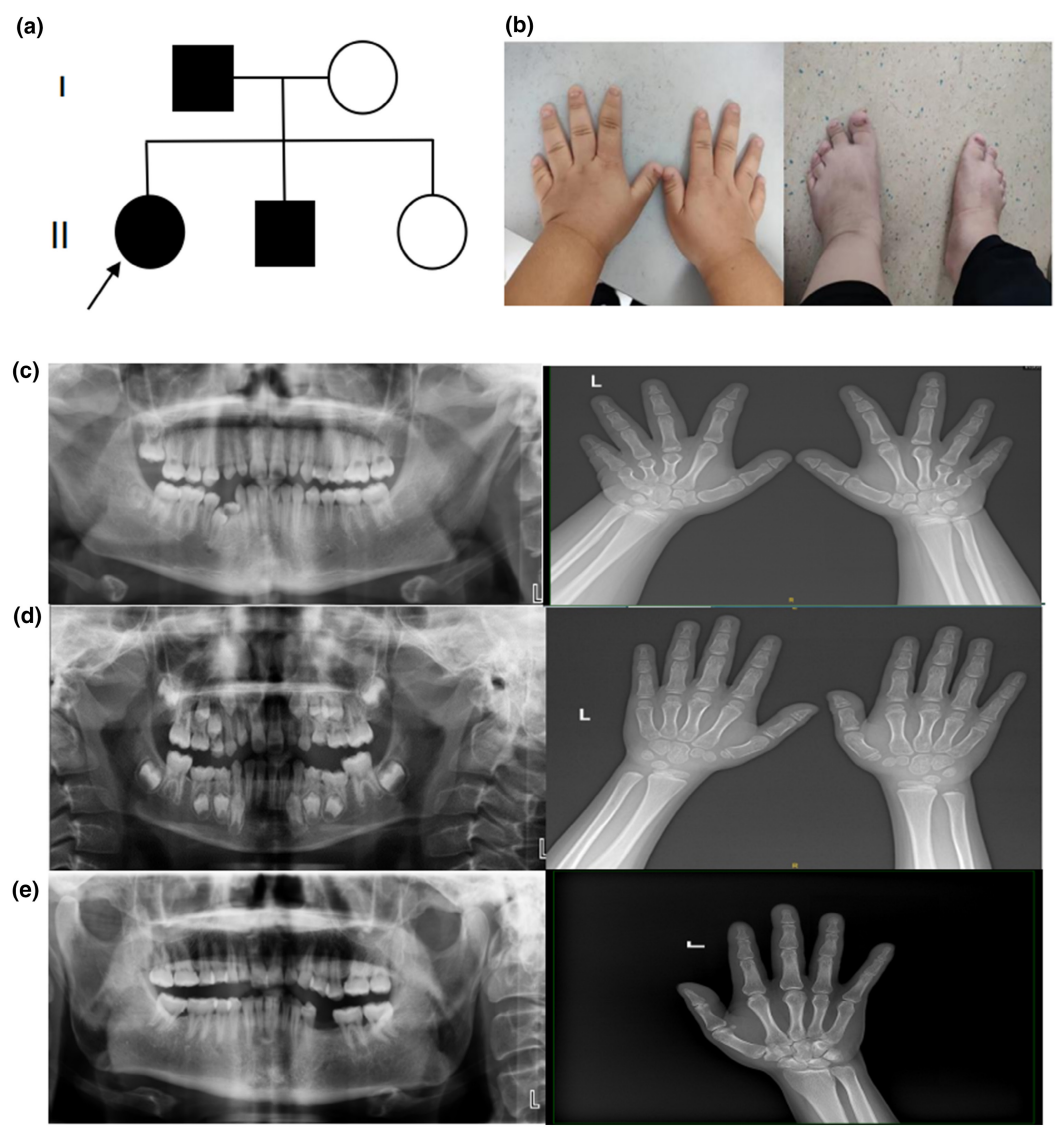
(a) Family tree: An arrow indicates the proband, filled symbols indicate affected individuals, circles denote females, and squares denote males. (b) Limb features of the proband. Oral and hand radiographs of individuals carrying heterozygous *PTH1R* variants: the proband (c), her brother (d), and her father (e).
